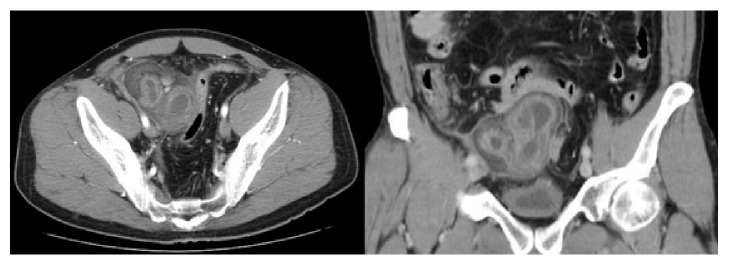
A computed tomography (CT) image revealed irregular polycystic mass connected to small intestine measuring 7.5 cm in a diameter in the lower abdomen.
